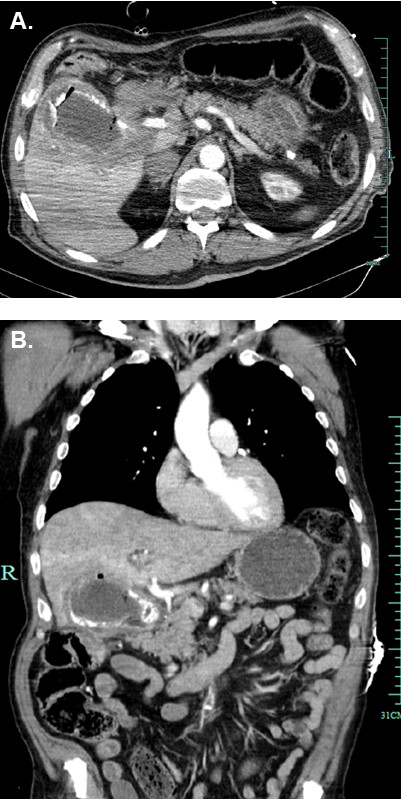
Initially performed trauma computer tomography body scan. (A) Axial intravenous contrast-enhanced CT scan and (B) coronal reformatted image of the anterior abdomen demonstrate a huge gallstone and an upper-abdominal process, which raised the suspicion of a perforated gallbladder.
